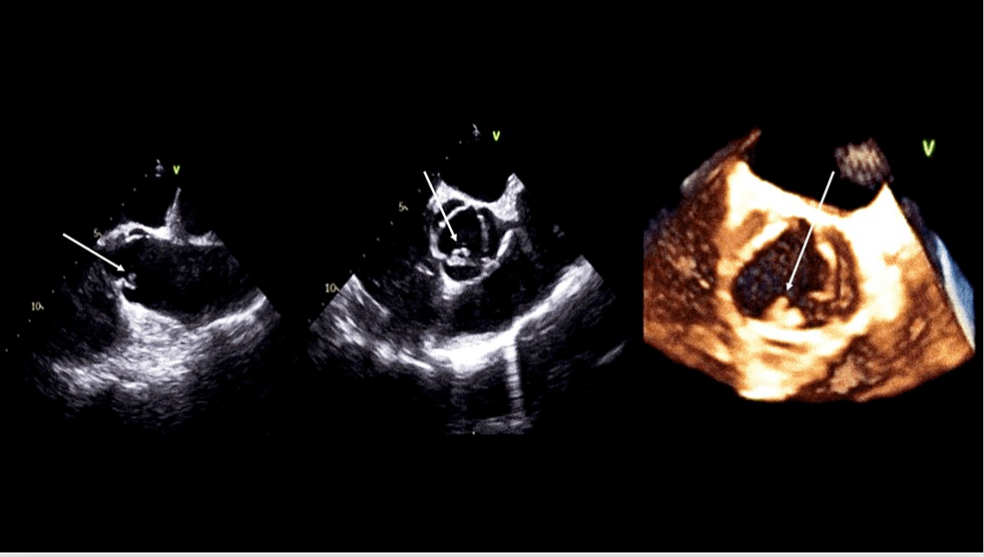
TEE images showing vegetation in the AV. AV, aortic valve; TEE, transesophageal echocardiography
